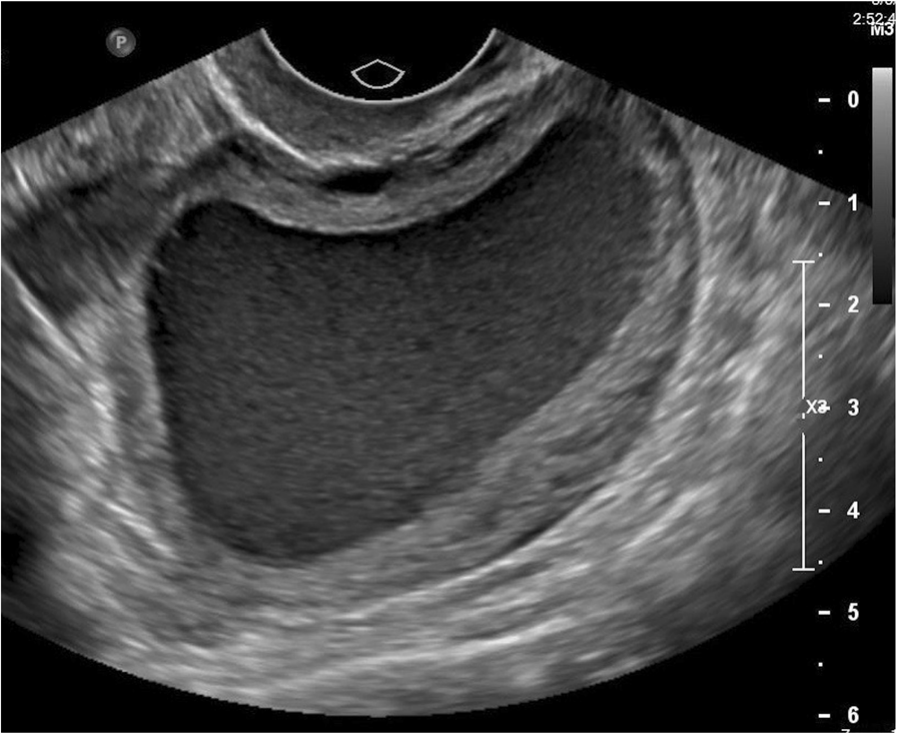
Transvaginal ultrasound. Uterus measuring 7.1×4.6×5.4 cm. The endometrium is distended with homogenous echogenic fluid
